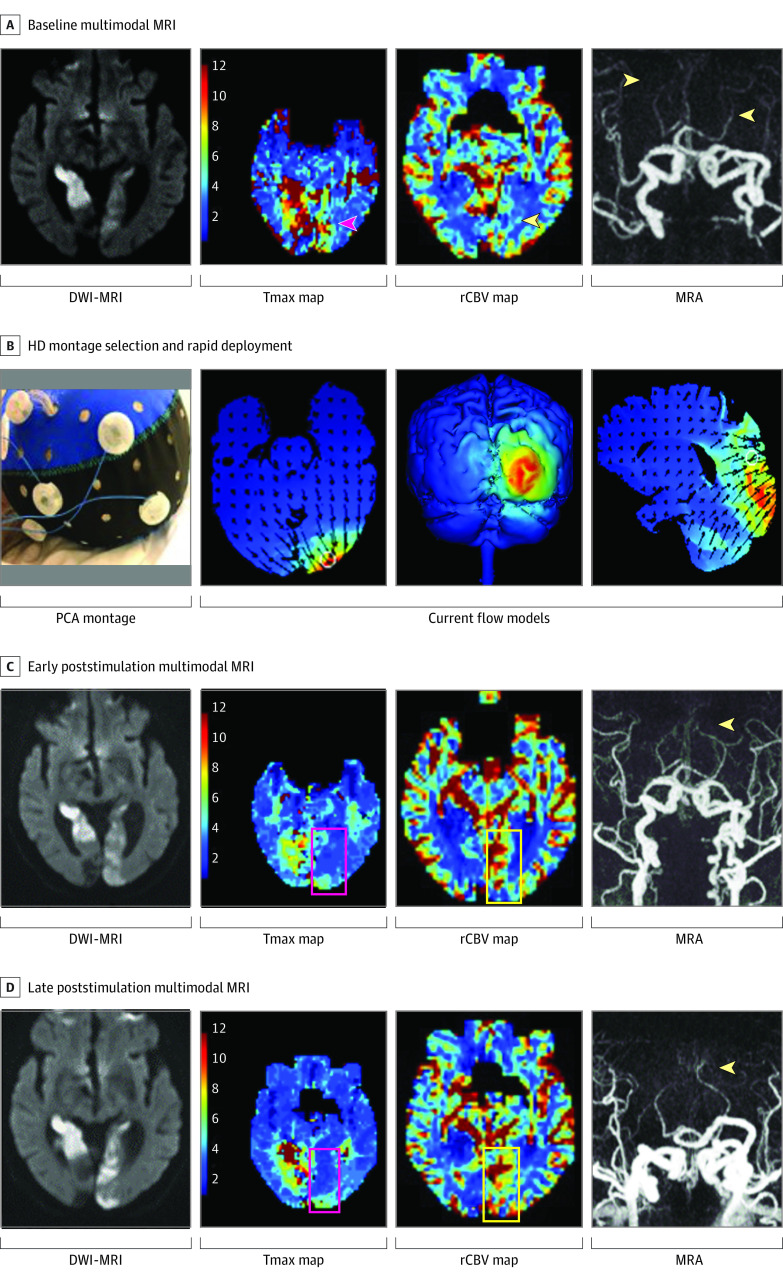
First Exemplar of a Transcranial Electrical Stimulation in Stroke Early After Onset Clinical Trial Patient Patient 1 was a male in his 70s with acute ischemic stroke due to bilateral posterior cerebral artery (PCA) occlusions. Given the symptomatic nature of the left PCA occlusion, he received 20 minutes of 1 milliamp (mA) high-definition (HD) cathodal transcranial direct current stimulation to the left occipital region only. Early alleviation of hypoperfusion, enhancement of relative cerebral blood volume, and recanalization were only observed on the stimulated side (left occipital lobe–left PCA territory). The contralateral right side, an internal control, remained ischemic due to persistent occlusion, highly suggestive of a true biological effect of stimulation. A, Acute infarctions of bilateral occipital regions (diffusion-weighted image [DWI] hyperintensities); hypoperfusion of bilateral PCA territories (time-to-maximum [Tmax] >6 seconds); symmetric bilateral relative cerebral blood volume (rCBV); bilateral PCA occlusions on MRA. B, A selection of PCA HD montage with current models predicting the e-field concentration over the ischemic region. C and D, Evolving bilateral occipital lobes acute infarcts (DWI hyperintensities); early and sustained alleviation of left occipital hypoperfusion; early and sustained rCBV enhancement of left occipital region; early and sustained left PCA partial recanalization. MRA indicates magnetic resonance angiography; MRI, magnetic resonance imaging.
